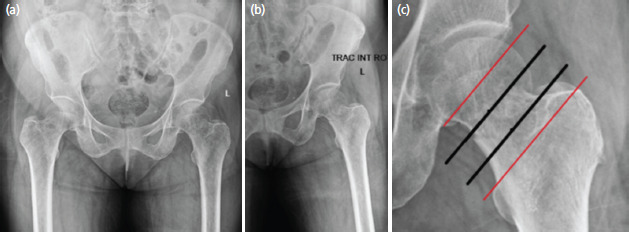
(a, b, c) Radiographs depicting 66-year-old female with Singh index grading 4 who sustained a transcervical fracture.
